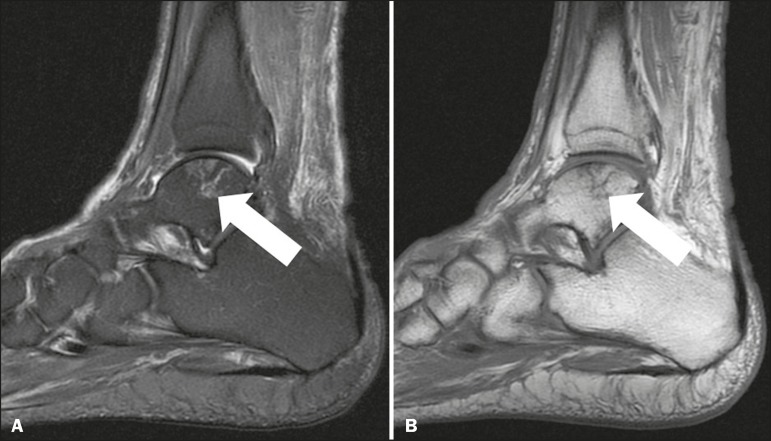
T1-weighted and fatsaturated T2-weighted MRI sequences showing an
